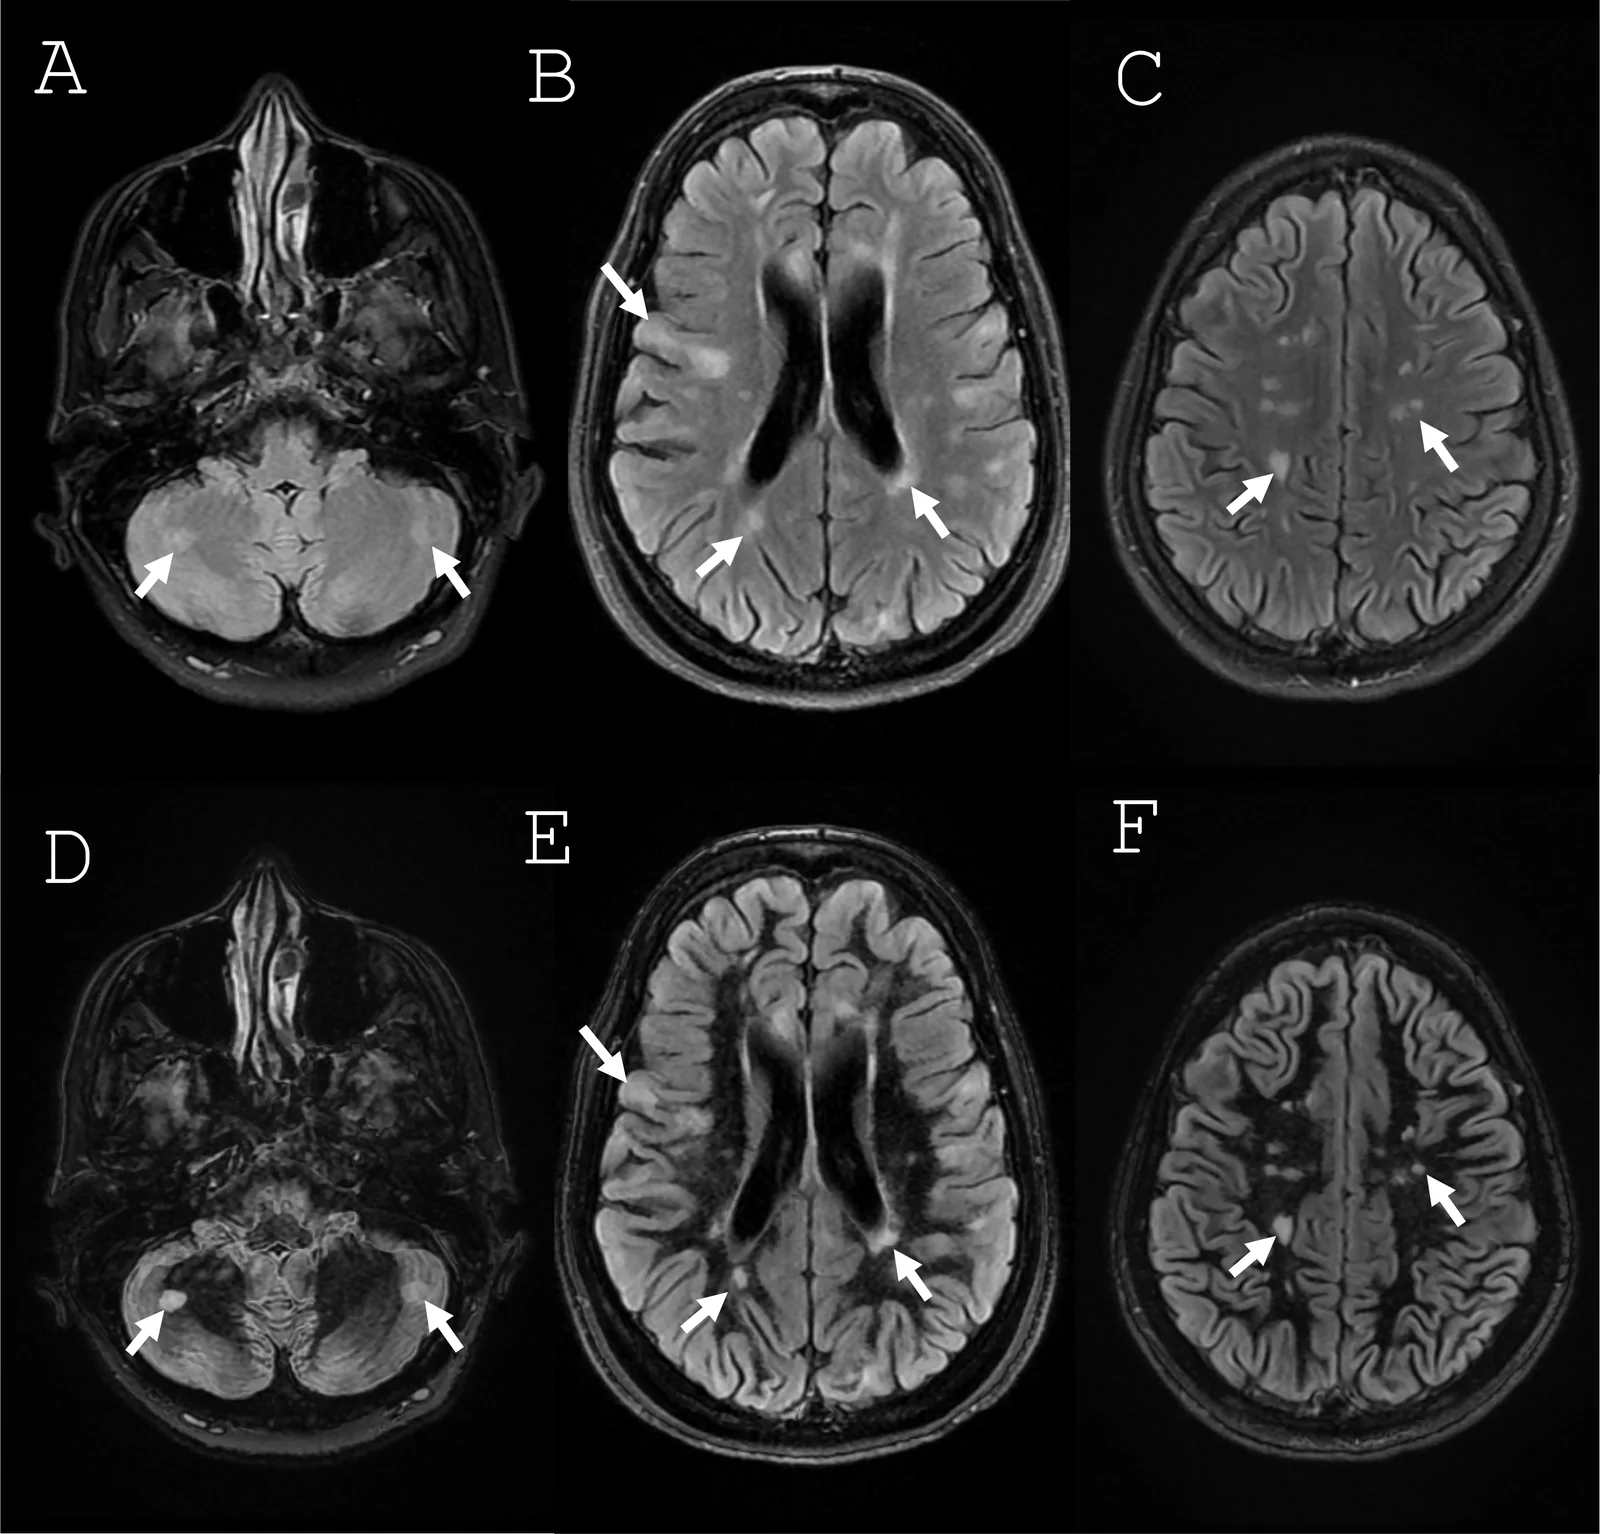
Axial brain MRI at the level of the posterior fossa (A&D), lateral ventricles (B&E), and centrum semiovale (C&F) for three different MS patients. Comparison of FLAIR images in the top row and MinPE FLAIR/WAIR images in the bottom row. Lesions marked with white arrows were brighter and more easily identifiable on MinPE FLAIR/WAIR than on FLAIR.
